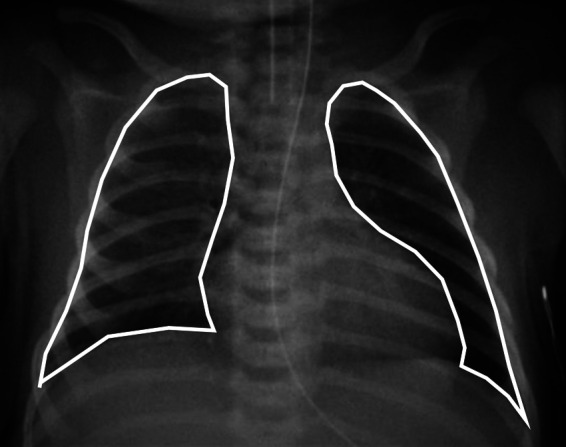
A chest radiograph of a term-born infant with poor perinatal adaptation on the first day of life. Method of free hand tracing of the perimeter of the CRTA excluding the mediastinal shadow.
